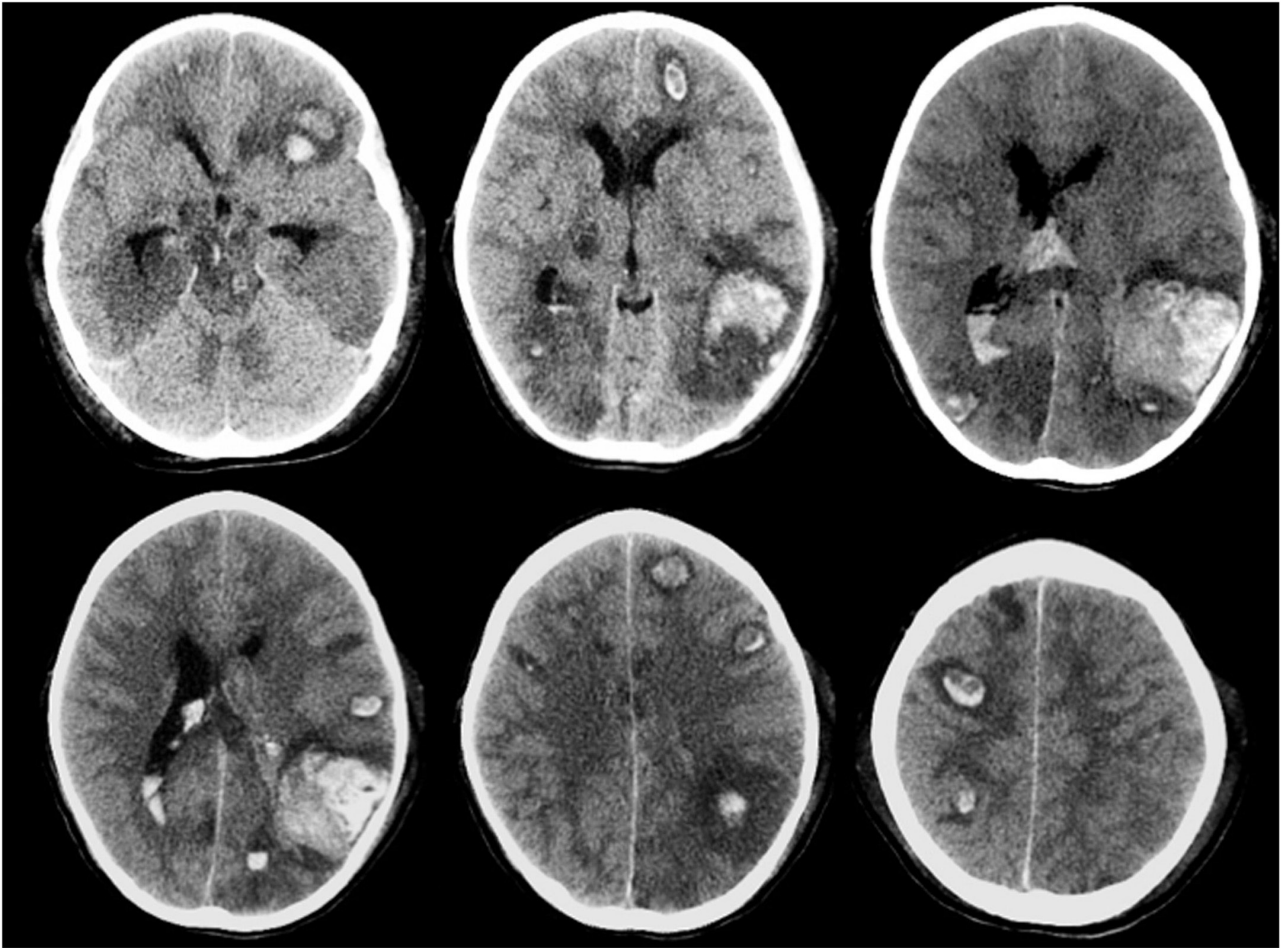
Multiple brain hemorrhage in a 12-year-old girl affected by acute myeloid leukemia. Axial computed tomography images show multiple hemorrhagic foci involving mainly the subcortical areas of the cerebral hemispheres, more evident in the left temporo-parieto-occipital region.
